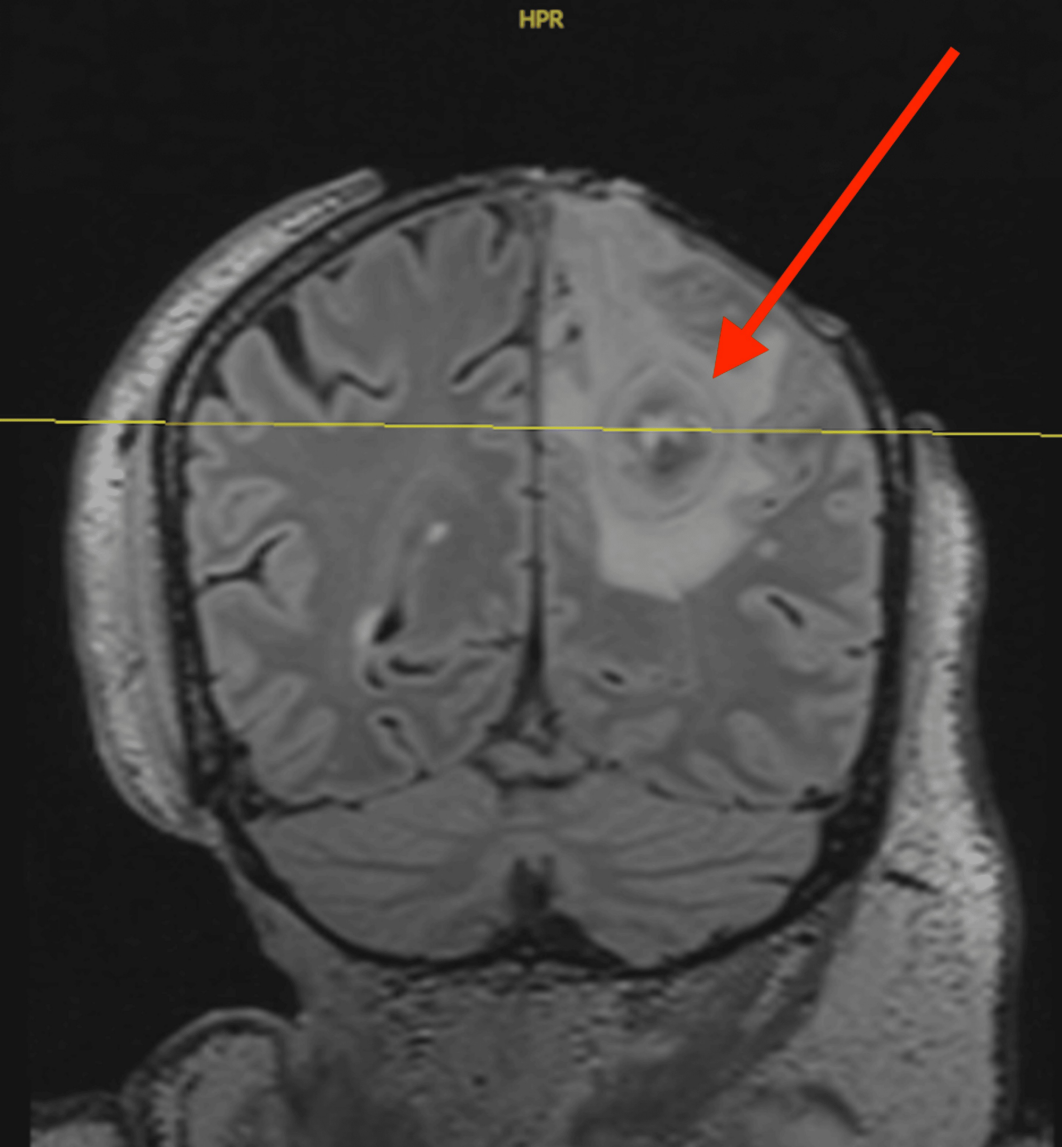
MRI Brain with and without contrast: Coronal FLAIR 3D MPR reformat image depicting large scalp defects in the frontoparietal vertex and right occipital regions, with irregularity of the underlying bone along the outer table. There are small areas of apparent loss of integrity in the inner table of the left parietal bone, as well as pus in the surgical resection cavity, located superior to the lesion described above, distributed across three different pockets. MRI study dated March 13, 2024. FLAIR: Fluid-attenuated inversion recovery; MPR: multiplanar reconstruction
